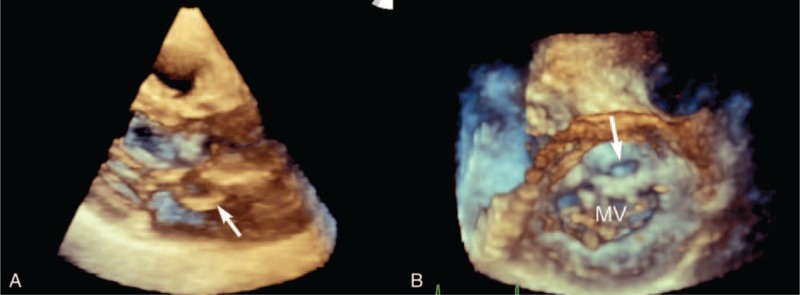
Nonstandard parasternal aortic valve short-axis view showing a pseudoaneurysm in the MAIVF region. (A) Two-dimensional echocardiography shows the expansion of the pseudoaneurysm during systole, protruding into the left and right atrium (star) and the communicating orifice between the lesion and the ascending aorta (arrow) with a diameter of 8 mm. (B) Color Doppler imaging shows the abnormal turbulence signal between the lesion and the ascending aorta (arrow). AAo = ascending aorta; LA = left atrium; MAIVF = mitral-aortic intervalvular fibrosa; P-MAIVF = pseudoaneurysm of the mitral-aortic intervalvular fibrosa; RA = right atrium; RV = right ventricle.
